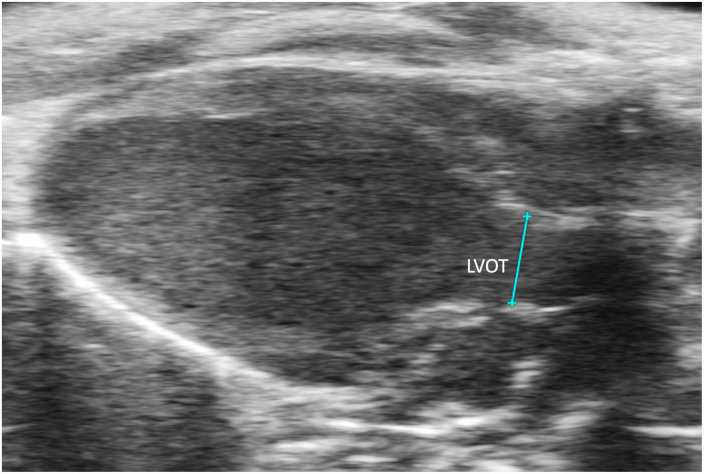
The left ventricular outflow tract (LVOT) length is acquired in the B-mode of the parasternal long axis view (PLAX).
